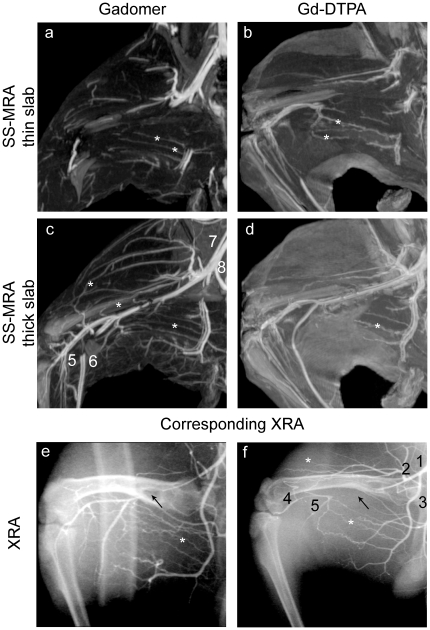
Contrast-enhanced MR angiograms with Gadomer and Gd-DTPA. Partial maximum intensity projections (MIPs) in the anterior-posterior direction of steady-state MR angiograms (SS-MRA) for Gadomer (left column) and Gd-DTPA (right column). On the thin-slab MIPs (thickness 3–4 slices, panels a and b), collaterals could be discerned for both contrast agents. Thick slab MIPs (>20 slices; panels c and d) show superior depiction of vessels with Gadomer. For Gd-DTPA, the images were blurred. Panels e and f show the corresponding x-ray angiograms (XRA). 1: femoral artery; 2: circumflex femoral artery; 3: deep femoral artery; 4: tibial artery; 5: popliteal artery; 6: popliteal vein; 7: iliac artery; 8: iliac vein. * indicate collaterals. The ligation is indicated with the arrow in the XRA images. All images were acquired on day 21.
